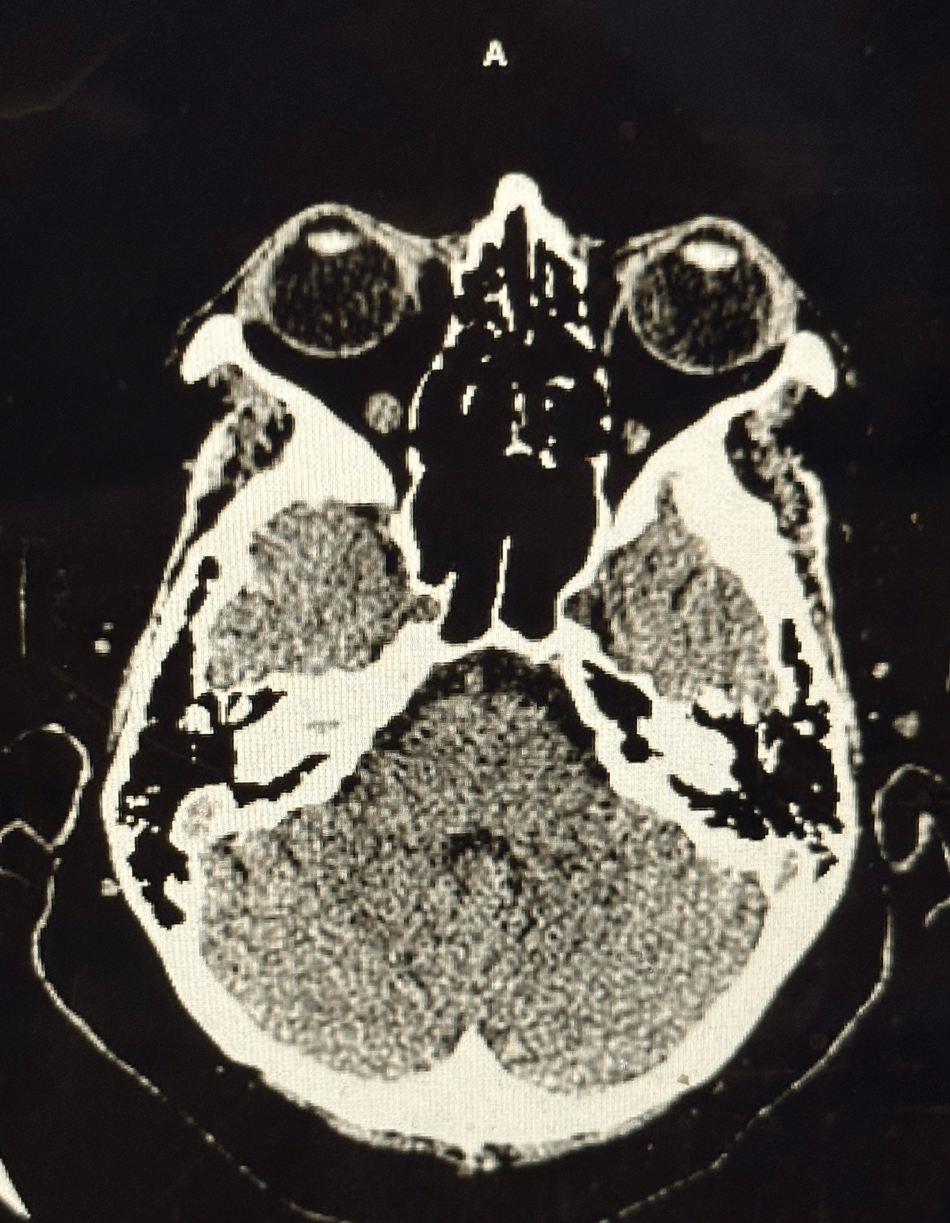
CT scan of the head or brain without contrast This image demonstrates no evidence of acute abnormalities as the cause of generalized weakness, tingling, and numbness.
